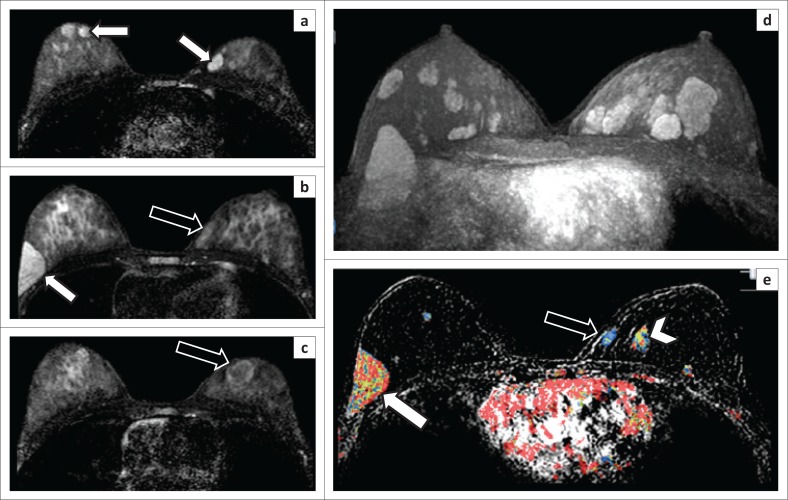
A breast magnetic resonance imaging of a patient with a total of 15 fibroadenomas demonstrating different magnetic resonance imaging appearances: (a–c) The T2-weighted fat saturation images demonstrate multiple homogeneously hyperintense circumscribed oval lesions (white arrows). Two circumscribed hypointense lesions (black arrows) can be seen in (b) and (c). In (d), a maximum intensity projection T1-weighted fat saturation post-contrast image, numerous breast masses are visualised. (e) The subtracted post-contrast T1-weighted fat saturation image with superimposed colour map demonstrates three lesions with different enhancement curves. One lesion with a predominant washout curve (white arrow), a lesion with a persistent enhancement curve (black arrow) and a lesion with mixed enhancement curve (arrowhead).
